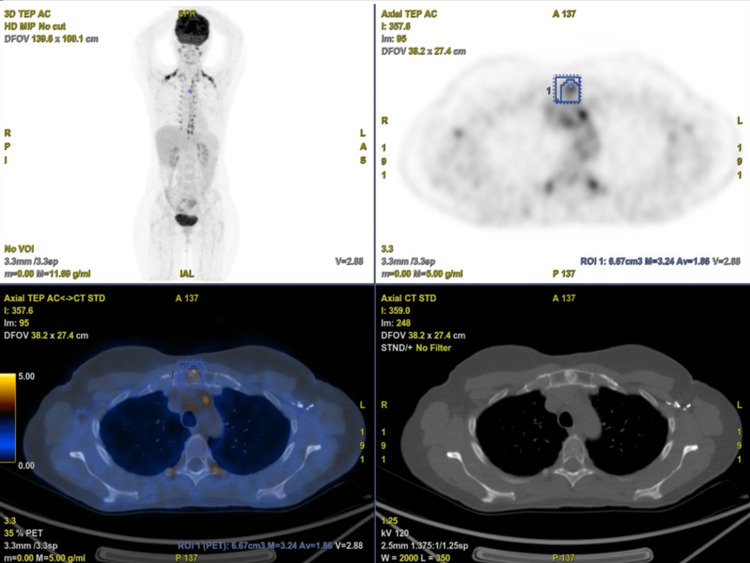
A FDG-PET scan demonstrates mild uptake with a maximum standardized uptake value (SUVmax) of 3.2. FDG-PET: fluorodeoxyglucose-positron emission tomography
